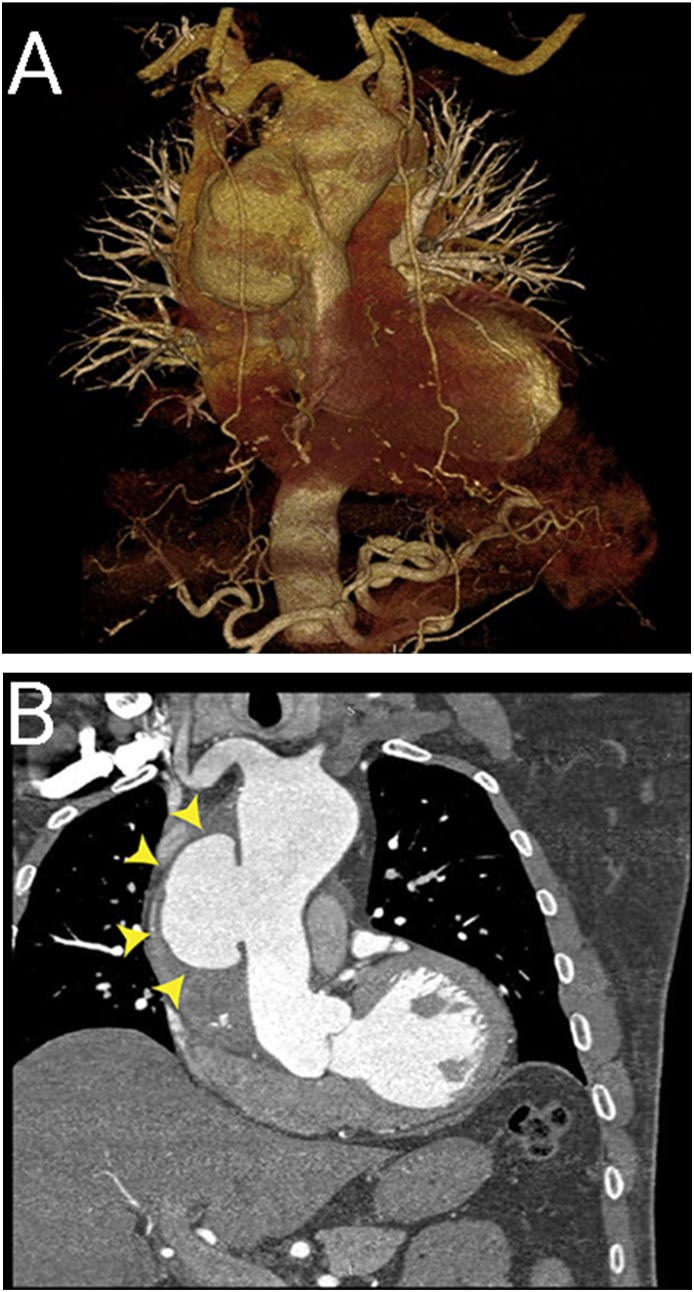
A. CT Angiography and B. Contrast enhanced CT scan showing a large dissected saccular aneurysm (yellow arrowheads). (For interpretation of the references to colour in this figure legend, the reader is referred to the Web version of this article.)
